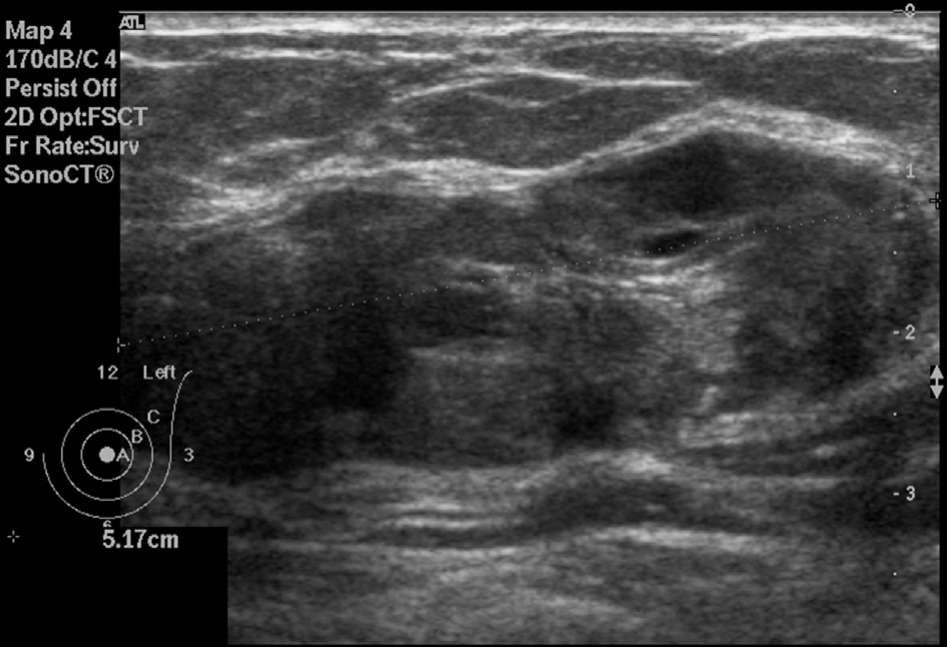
Ultrasound of the left breast centered on the palpable lump showing an oval, heterogeneous lesions with smooth borders.
